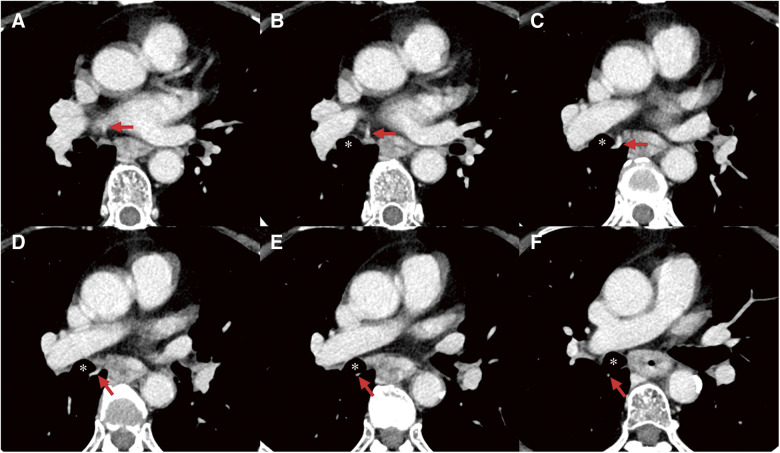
CT imaging of the course of the anomalous RSPPV, V2. (A, B) Anomalous RSPPV draining into RSPV. (C–F) Anomalous RSPPV (arrows) running dorsal side of the right intermediate bronchus. CT: computed tomography; RSPPV: right superior posterior pulmonary vein; RSPV: right superior pulmonary vein. *Right main bronchus
